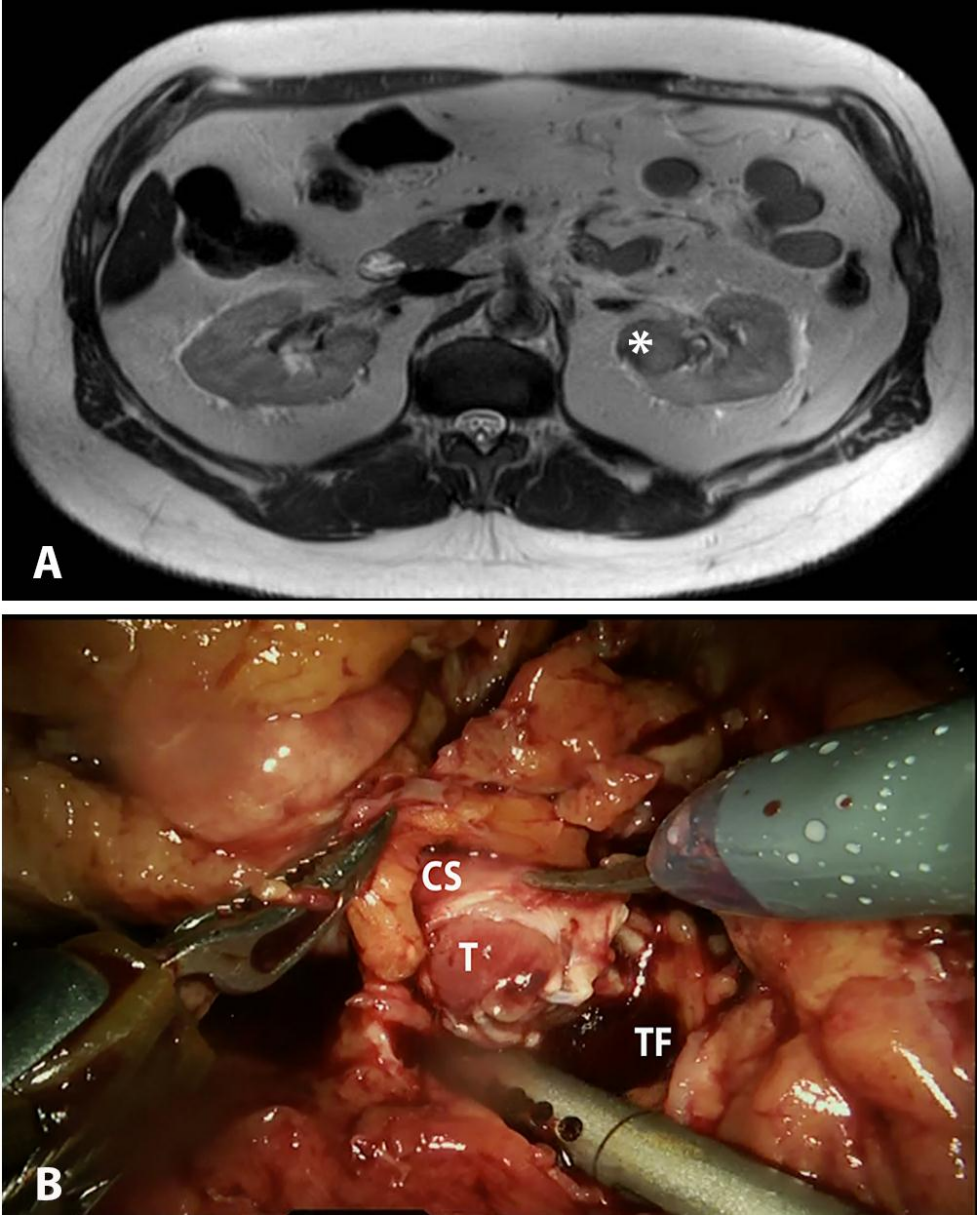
A, Magnetic resonance imaging (MRI) showing endophytic, posterior upper pole left renal mass (indicated by *), suspicious for carcinoma. B, Intraoperative photograph showing grossly invasive mass involving the lumen of the collecting system. T – Tumor; CS – collecting system; TF – tumor fossa.
